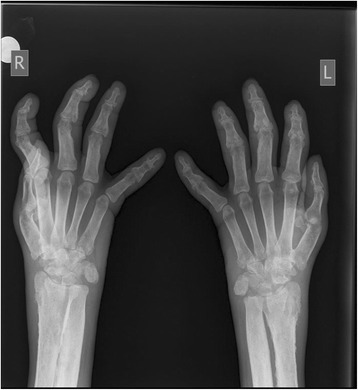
X-rays of hands showing expansion of ulna shaft and thickening of second to fourth digits
